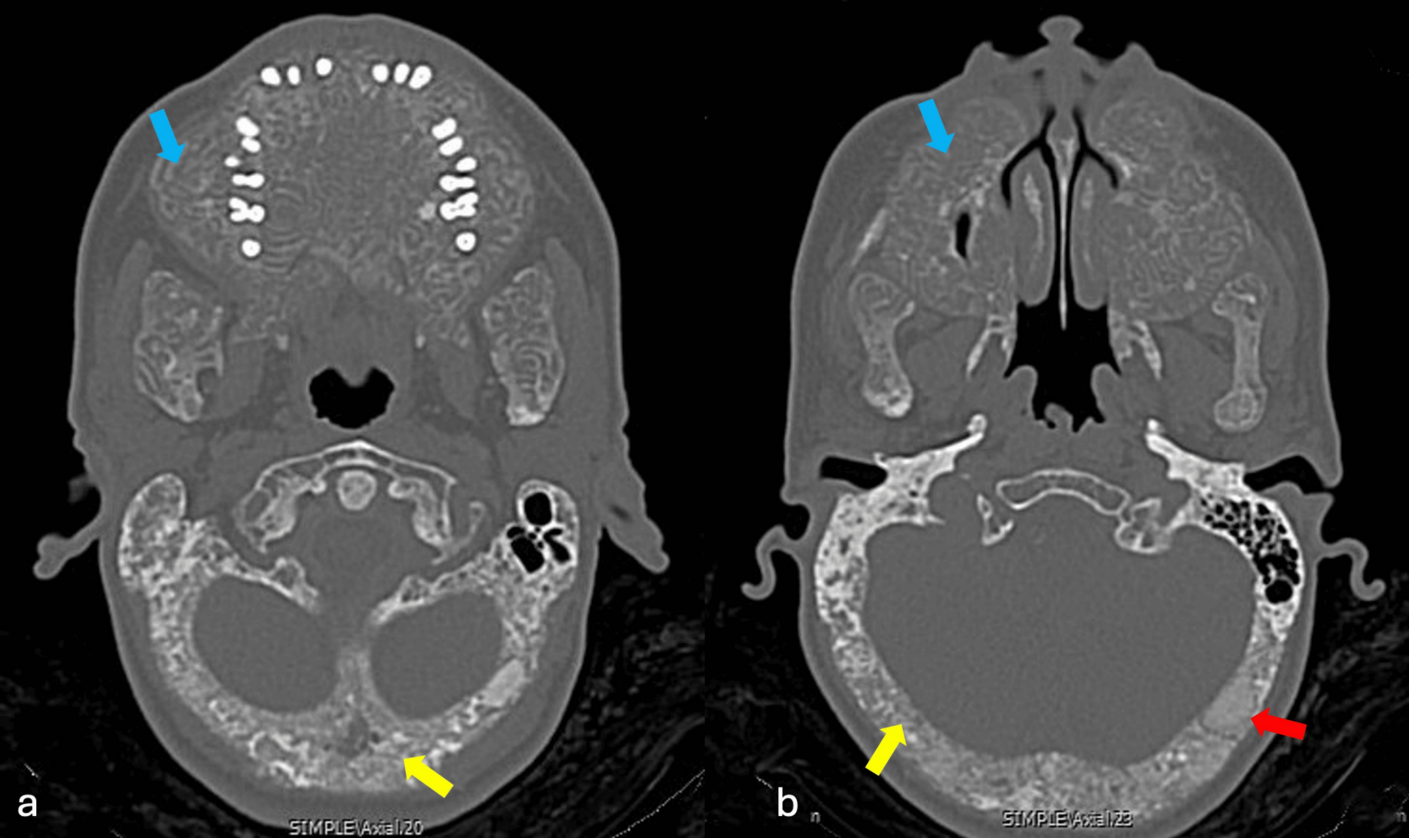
CT of the skull in bone window axial images. (A, B) Diffuse craniofacial bone thickening and deformity, with marked maxillary and mandibular overgrowth with significant cortical expansion (blue arrow), diffuse “salt and pepper” appearance predominantly in the calvaria and skull base (yellow arrow), and rounded focal ground-glass regions within the calvarium (red arrow).
